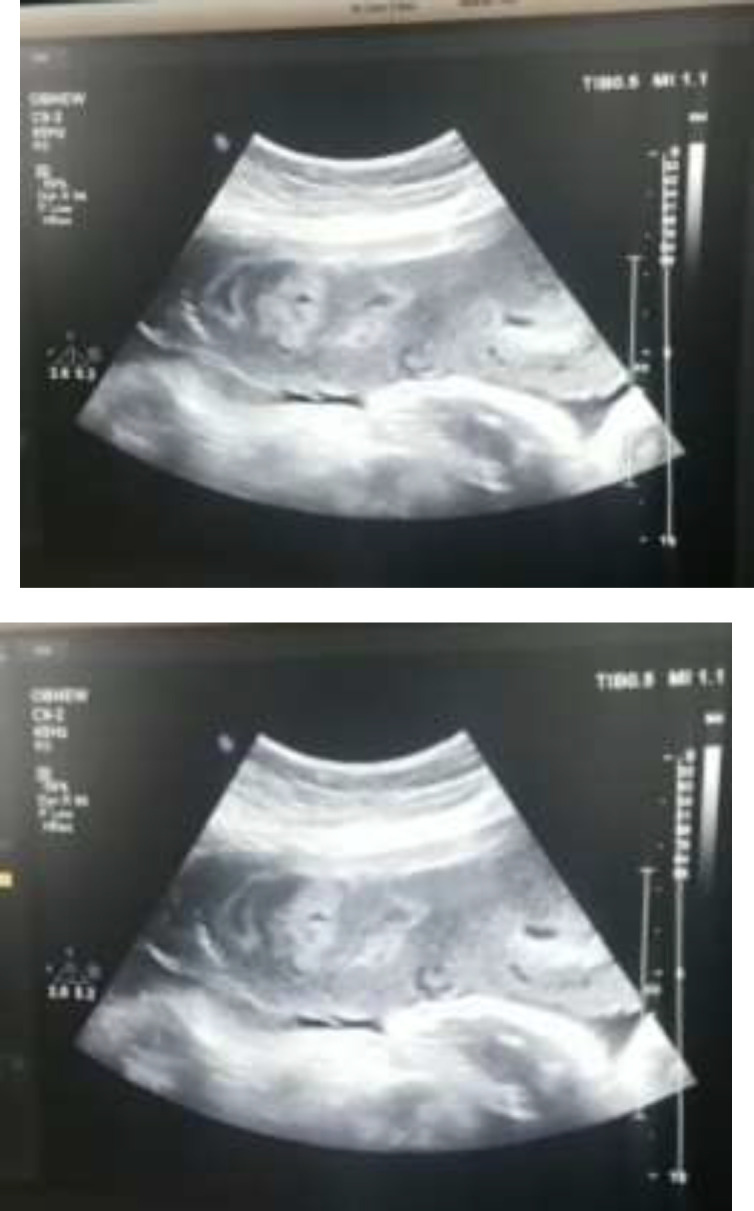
Ultrasound images of the placenta showing damage and fragmentation
